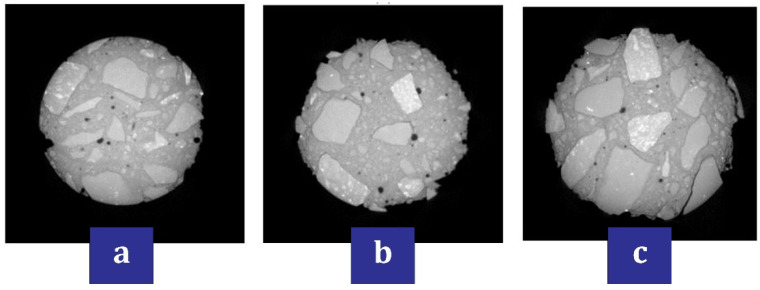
Appearance (CT scanning) of the mixture containing 0.6% nano-TiO_2_: (a) 25 cycles, (b) 50 cycles, and (c) 75 cycles [126].
